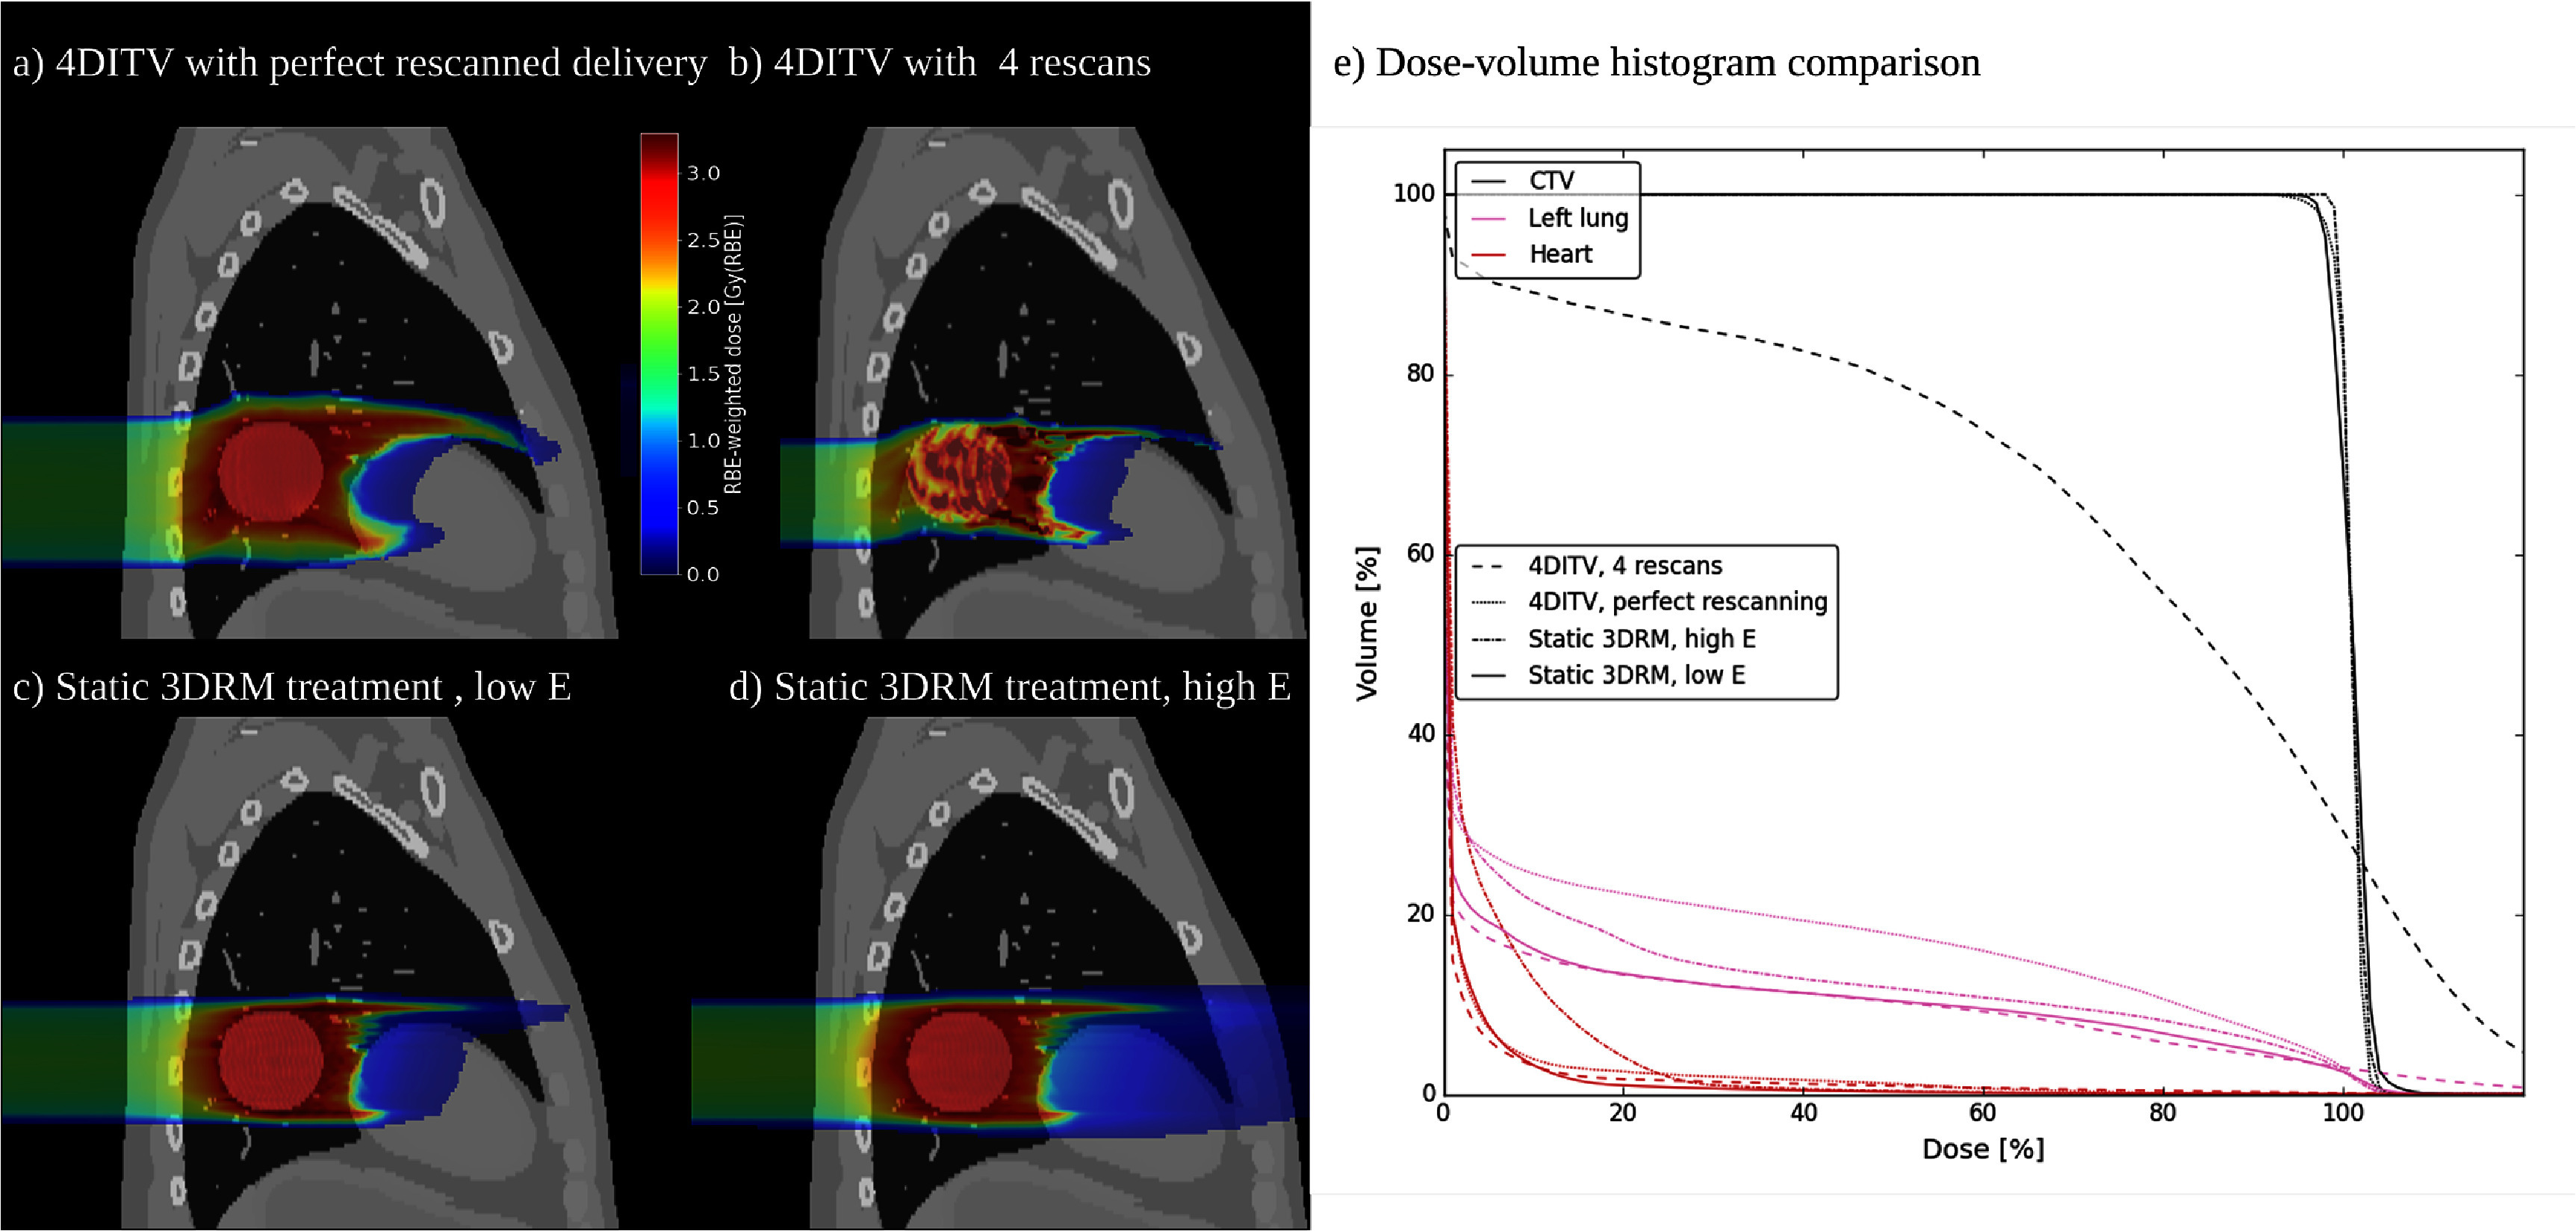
Dosimetric analysis of the benefit of a static 3DRM treatment versus an ITV treatment. (a)–(d): Comparison of dose slices overlaid on the XCAT CT reference phase. (a) and (b) were computed on the full 4DCT of the patient with perfect and realistic rescanning respectively. (c) and (d) are static 3DRM treatments on the reference phase assuming active and passive energy switching respectively. In active switching, the beam energy was chosen to be equal to the maximum range of the target whereas for passive, the beam energy was changed with a 120 mm WET bolus in front of the patient, with the beam energy kept at 350 MeV/u.
